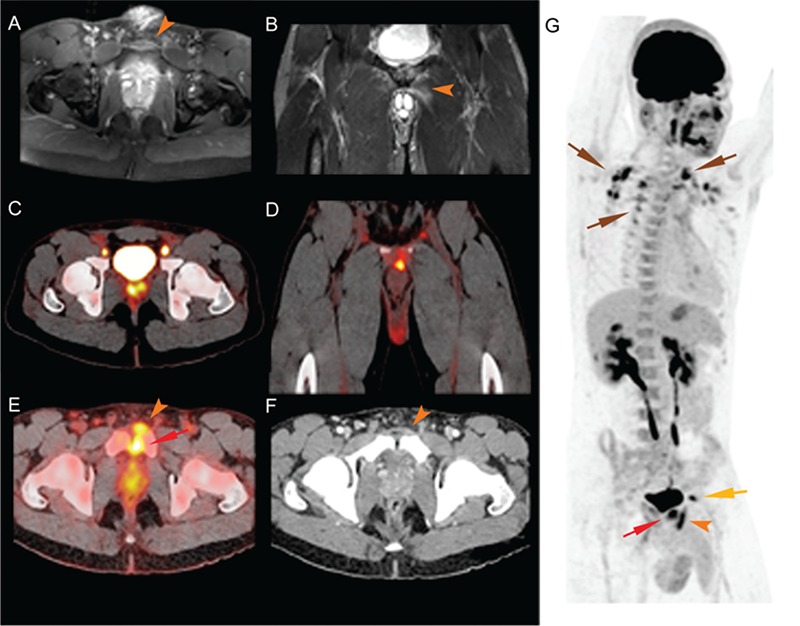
We present the case of a 23-year-old male African native patient presenting with fever, lumbalgia and dysuria after returning from a trip to Togo. The patient is a professional athlete (soccer player) with a known history of malaria during childhood. On palpation, there was pain over the pubic tubercle and the digital rectal exam was tender and sensitive. The blood formula was normal except elevated [C-reactive protein (CRP): 118 mg/L]. Recurrence of malaria had been excluded by repeated thick blood smears. Both blood and urinary cultures were positive for methicillinresistant *Staphylococcus aureus*. Computed tomography (CT) with nephrographic contrast and dedicated ultrasound ruled out pyelonephritis. A pelvic magnetic resonance imaging (MRI) was performed to rule out arthritis and osteomyelitis that revealed a thickening of the aponeurosis of the left rectus abdominis muscle on T1-weighted axial sequence after injection of gadolinium (A, arrow head), a hyper-signal of the symphysis on the STIR-weighted sequence corresponding to marrow edema without articular effusion, and a hyper-signal corresponding to a strain of the left adductor longus muscle (B, arrow head) characteristic of athletic pubalgia (1,2). ^18^F-FDG positron emission tomography/CT (PET/CT) found an increased prostatic tracer uptake along with bilateral external iliac lymph nodes hyper-metabolism (C), and also showed hyper-metabolism of the insertion of the left longus adductor (D) and of the left rectus abdominis (E and G, orange arrow) with a focal uptake in the pubic symphysis (E and G, red arrow) that were in concordance with the MRI findings. Increased ^18^F-FDG uptake on the molecular inversion probe sequence (G) in the supraclavicular, latero-cervical and para-vertebral regions corresponded to activated brown adipose tissue (brown arrow), the yellow arrow corresponds to the left iliac node; the right iliac node and the prostate were masked by the bladder. The CT scan (F) with contrast media confirmed the findings (thickening of the aponeurosis of the left rectus abdominis, arrow head). A prostatic origin of the infection was presumed and antibiotic therapy was initiated (intravenous vancomycin, then co-trimoxazole per os). Regression of fever, normalization of CRP and clearing of the cultures were observed rapidly.
